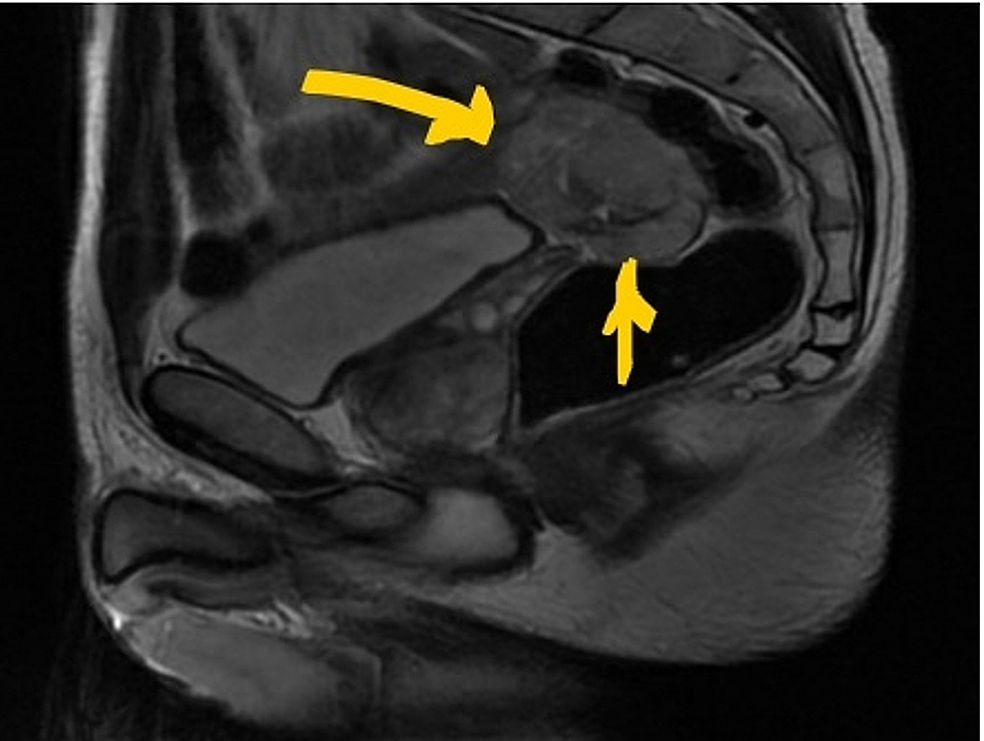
MRI image in the sagittal plane The image shows a soft tissue mass in the right pelvis, measuring approximately 7 cm. It is proximal to the urinary bladder and seminal vesicles and is unrelated to them (yellow arrows). It has heterogeneous enhancement. It is abutting the colon but does not involve the colon MRI: magnetic resonance imaging
